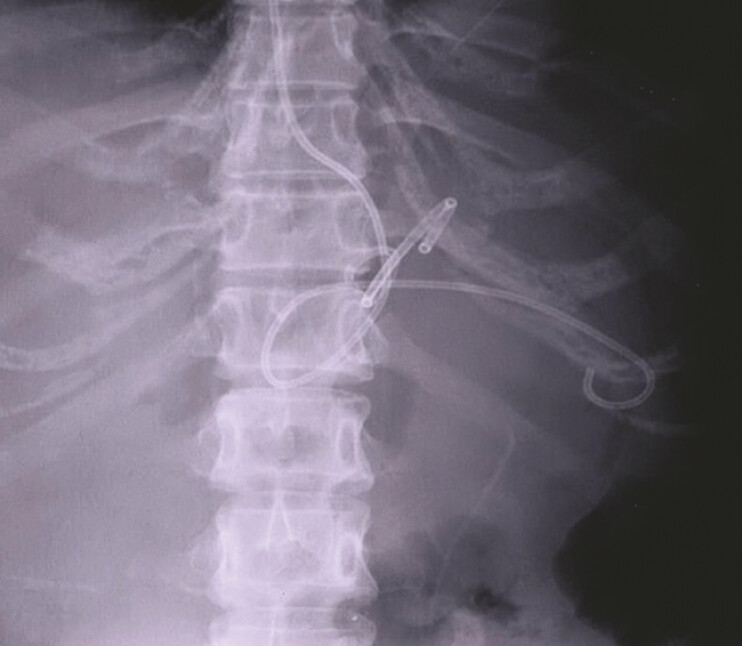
X-ray showed a stent and a nasocystic catheter.
